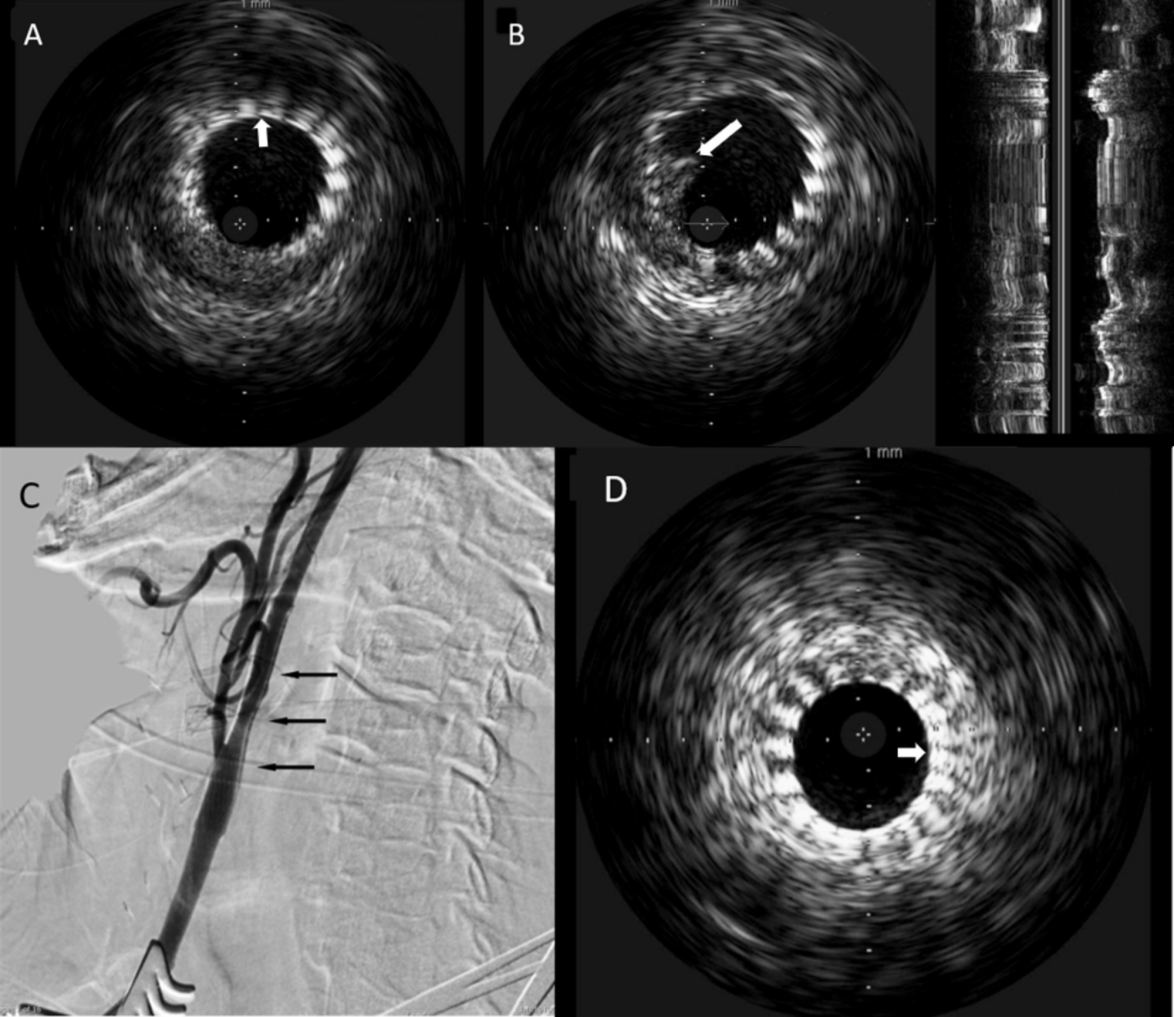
Poststenting intravascular ultrasound and digital subtraction carotid angiogram of Case 2 (A) Poststenting intravascular ultrasound image demonstrating good wall apposition of the stent (arrow). (B) Intraluminal debris noted inside stent (arrow). (C) Resolution of thrombus noted after placement of the second stent (arrow). (D) Final lateral projection diagnostic angiogram demonstrating significantly improved vessel caliber (arrows).
